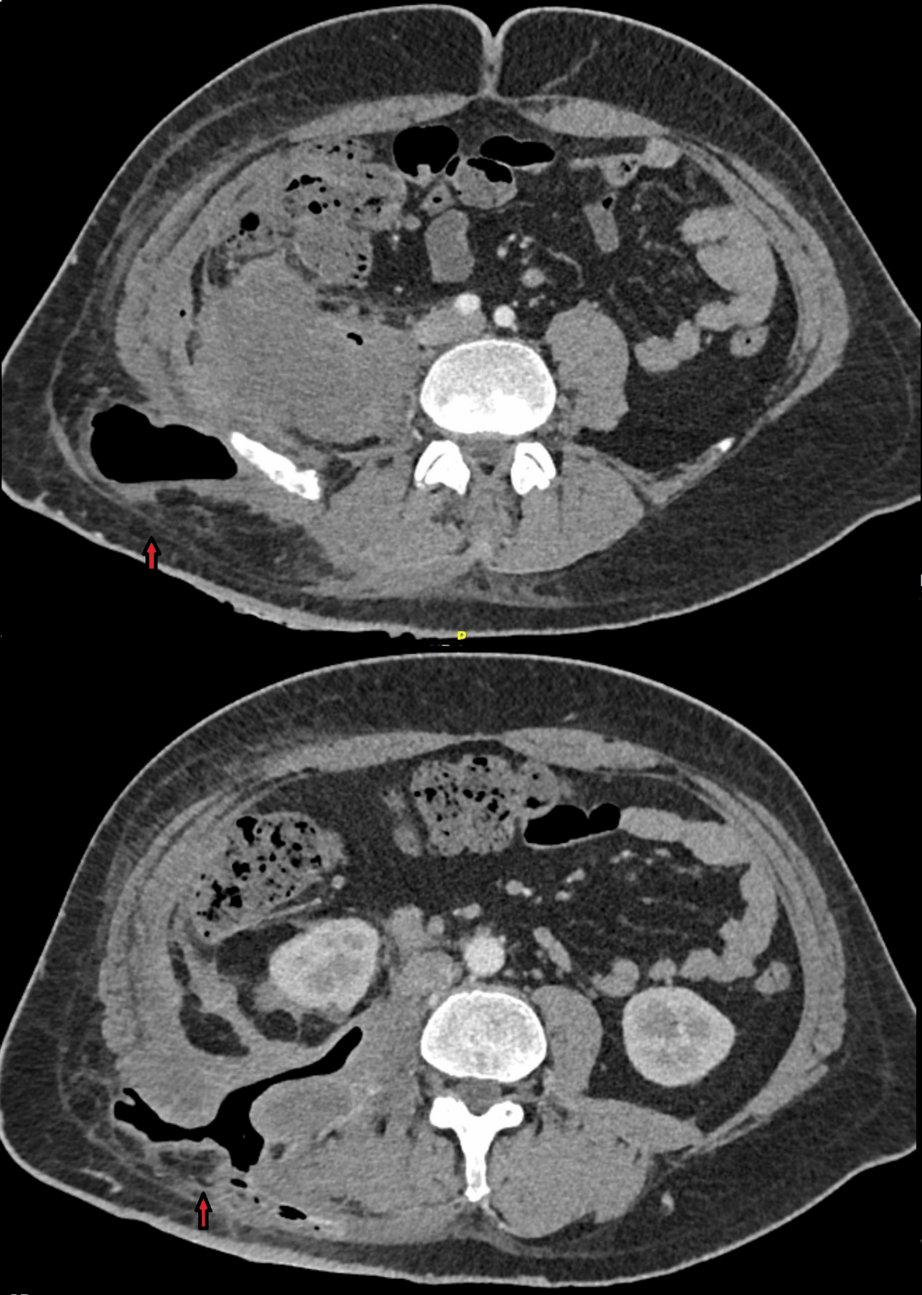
Postoperative CT findings revealing the drain track (red arrow).
